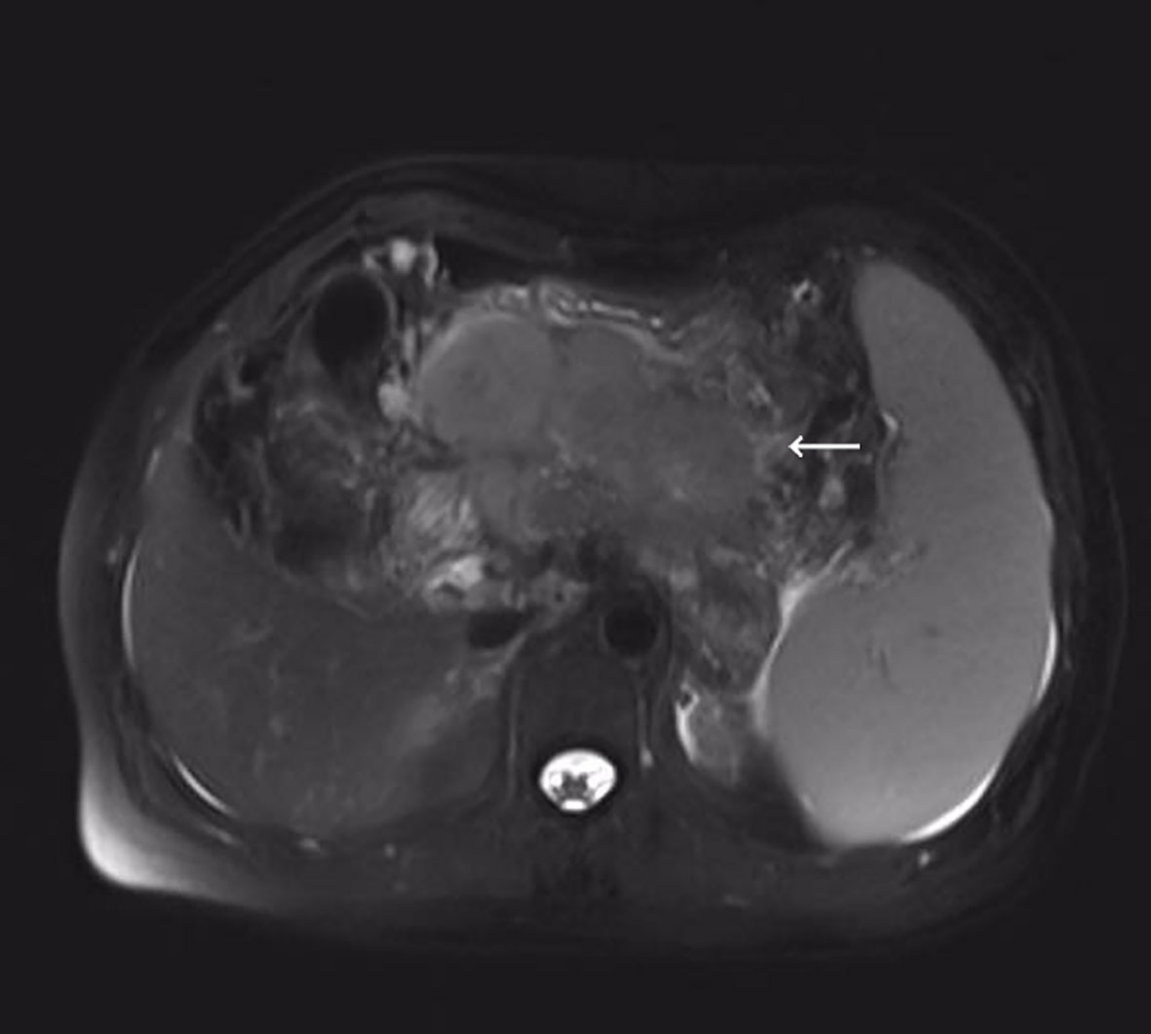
T2-weighted magnetic resonance imaging (MRI) showed irregular pancreatic mass measuring 105 mm in the maximum diameter.
